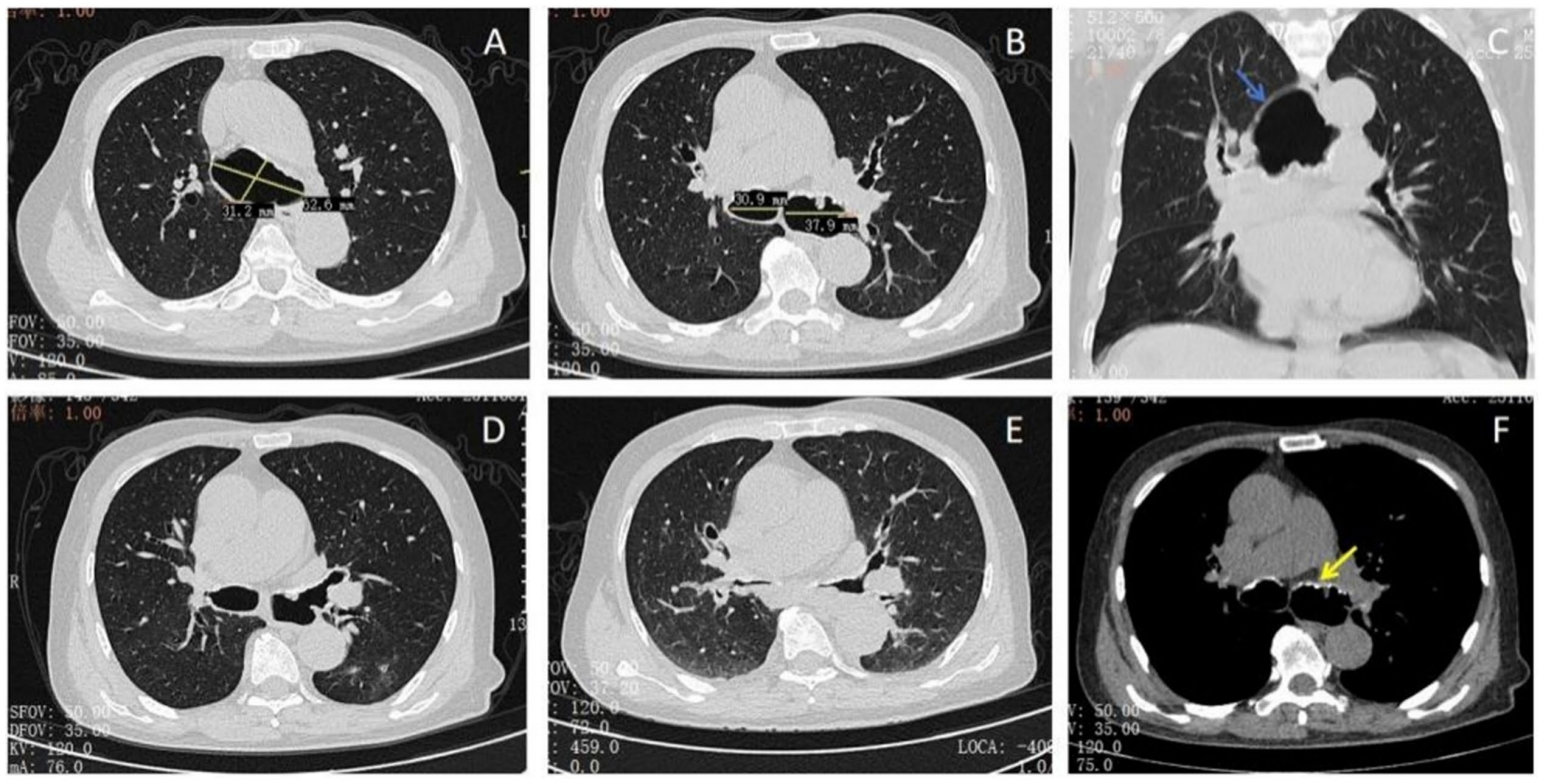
Thoracic computed tomography: (A) dilation of the trachea (62.6*31.2 mm). (B) Dilation of the main bronchi shown above. (C) The muscular layer of the tracheal wall was evidently thinner (blue arrow). (D,E) The constriction of the main bronchi was more than 50% during expiration. (F) CT images showed irregular submucosal nodular calcification (yellow arrow).
